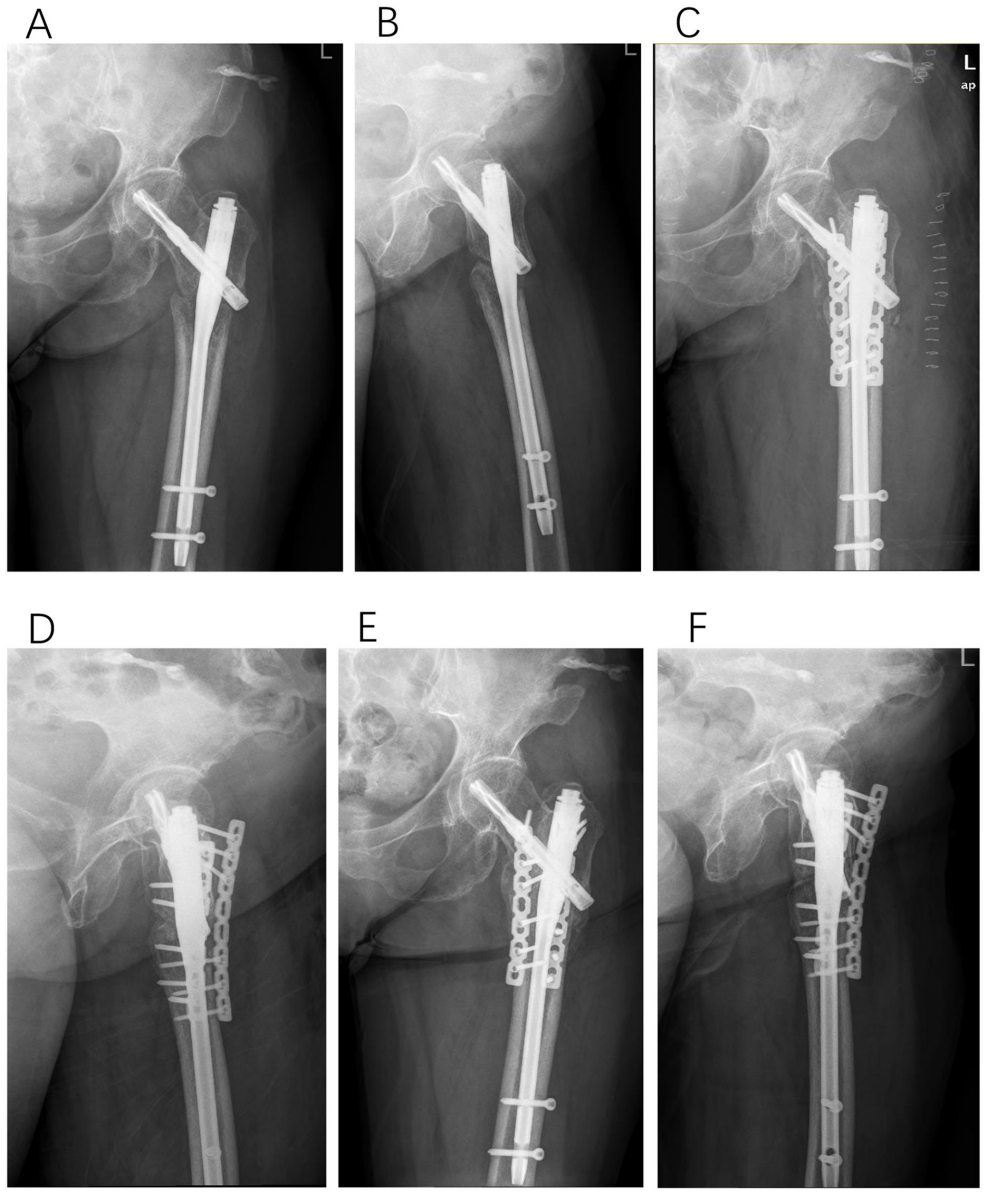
Type I, a 67-year-old female patient underwent PFNA fixation for a left intertrochanteric femoral fracture. Eight months post-operatively, radiographic evaluation revealed non-union at the fracture site. In the revision surgery, two auxiliary plates were added in conjunction with autologous bone grafting. Six months following this revision, successful bony union was achieved. (A, B) Anteroposterior and lateral X-rays of the femur obtained 8 months after initial PFNA fixation. (C, D) Anteroposterior and lateral X-rays of the femur immediately following the revision surgery. (E, F) Successful bony union confirmed 6 months post-revision surgery.
